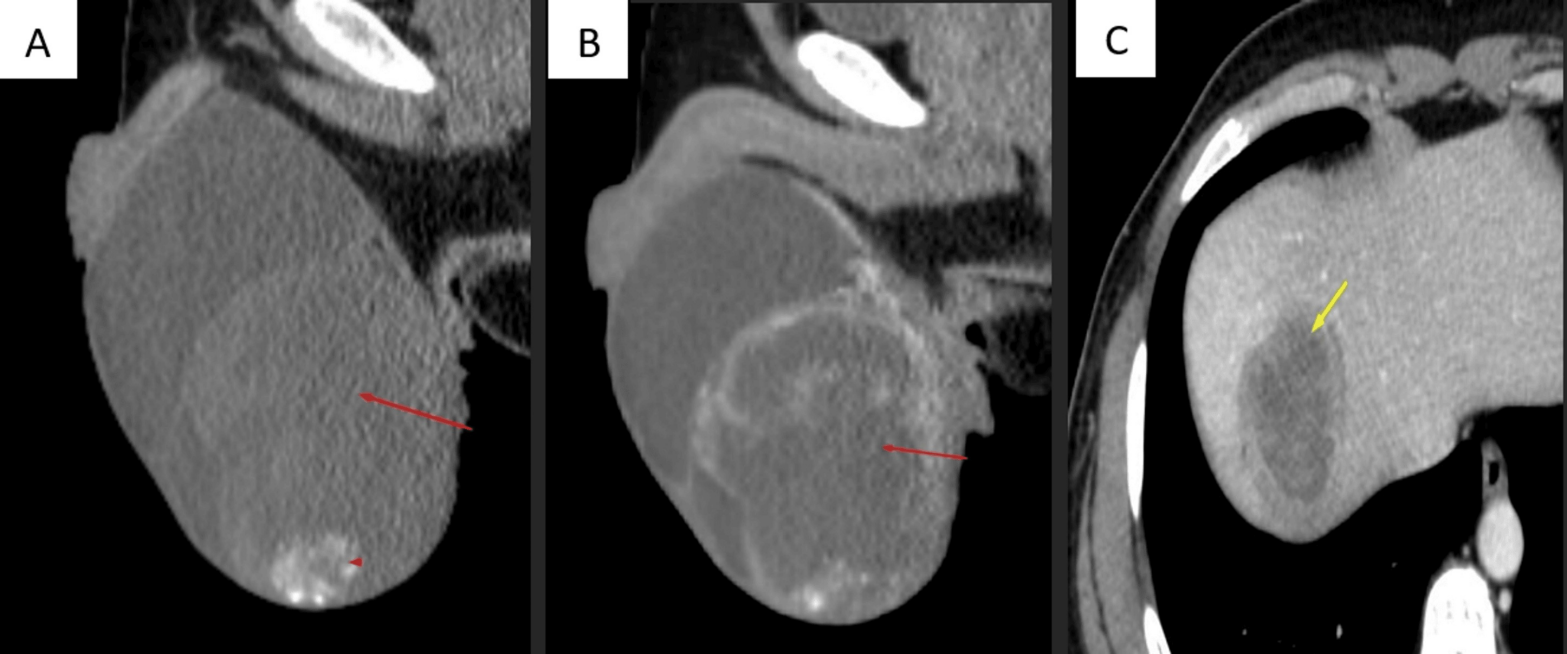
Unenhanced CT at sagittal plane, contrast-enhanced CT in venous phase at sagittal plane, and contrast-enhanced CT in venous phase at axial plane Unenhanced CT at sagittal plane showing enlarged testis with heterodense mass (red arrow) with calcific specks (arrowhead) in inferior aspect (A). Contrast-enhanced CT in venous phase at sagittal plane showing heterogenous contrast enhancement (red arrow) (B). Contrast-enhanced CT in venous phase at axial plane showing hepatic metastasis (yellow arrow) in right lobe of liver (C).
